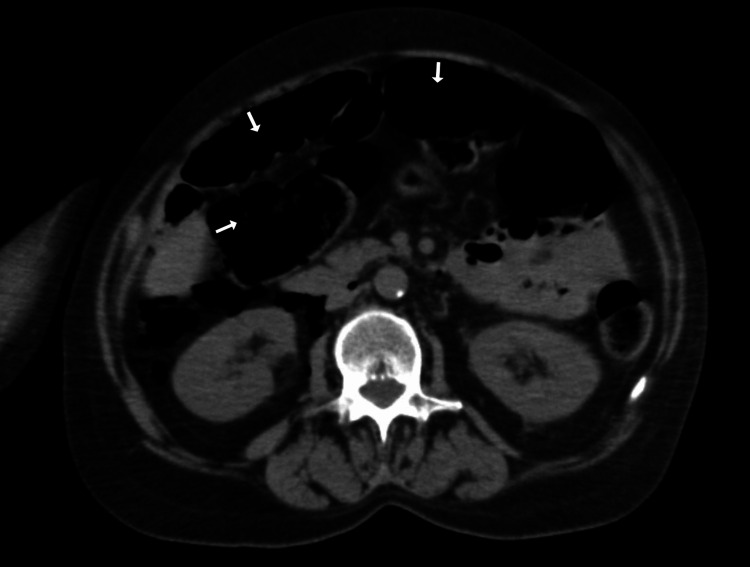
CT Scan Abdomen and Pelvis without contrast- Cross-sectional view with air in the peritoneum from Intestinal perforation.
